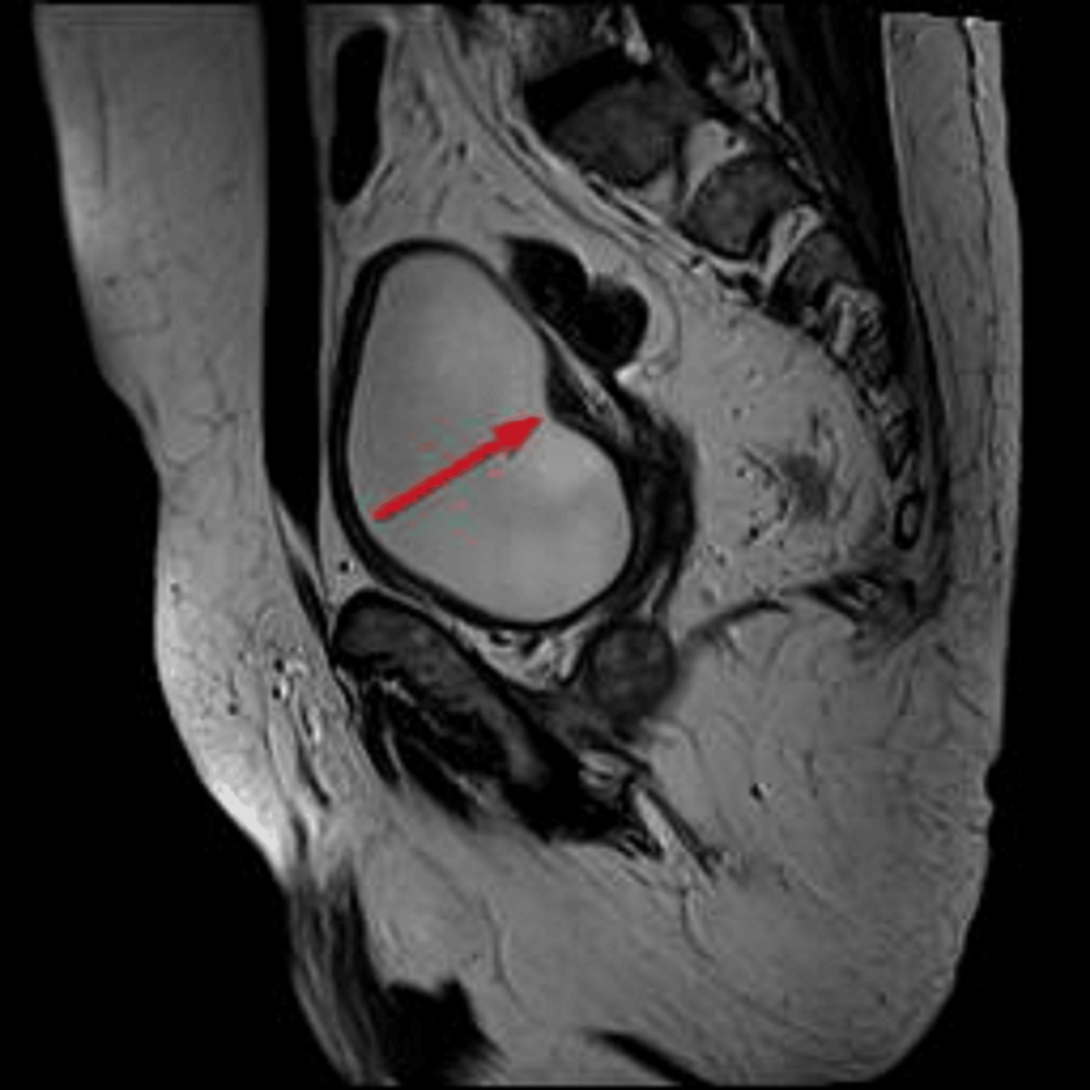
Resolved bladder wall lesion can be seen in MRI T2 sagittal images.
